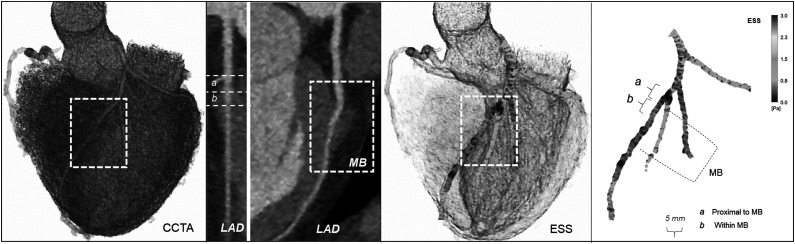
Endothelial shear stress (ESS)–profiling methodology. Coronary computed tomography angiography multiplanar reconstruction images and cross-sectional views of the coronary arteries were created and interpreted. The coronary artery lumen was segmented and reconstructed in three-dimensional space and ESS was calculated with the use of computational fluid dynamics. Local ESS values were extracted for the coronary segments of interest.
